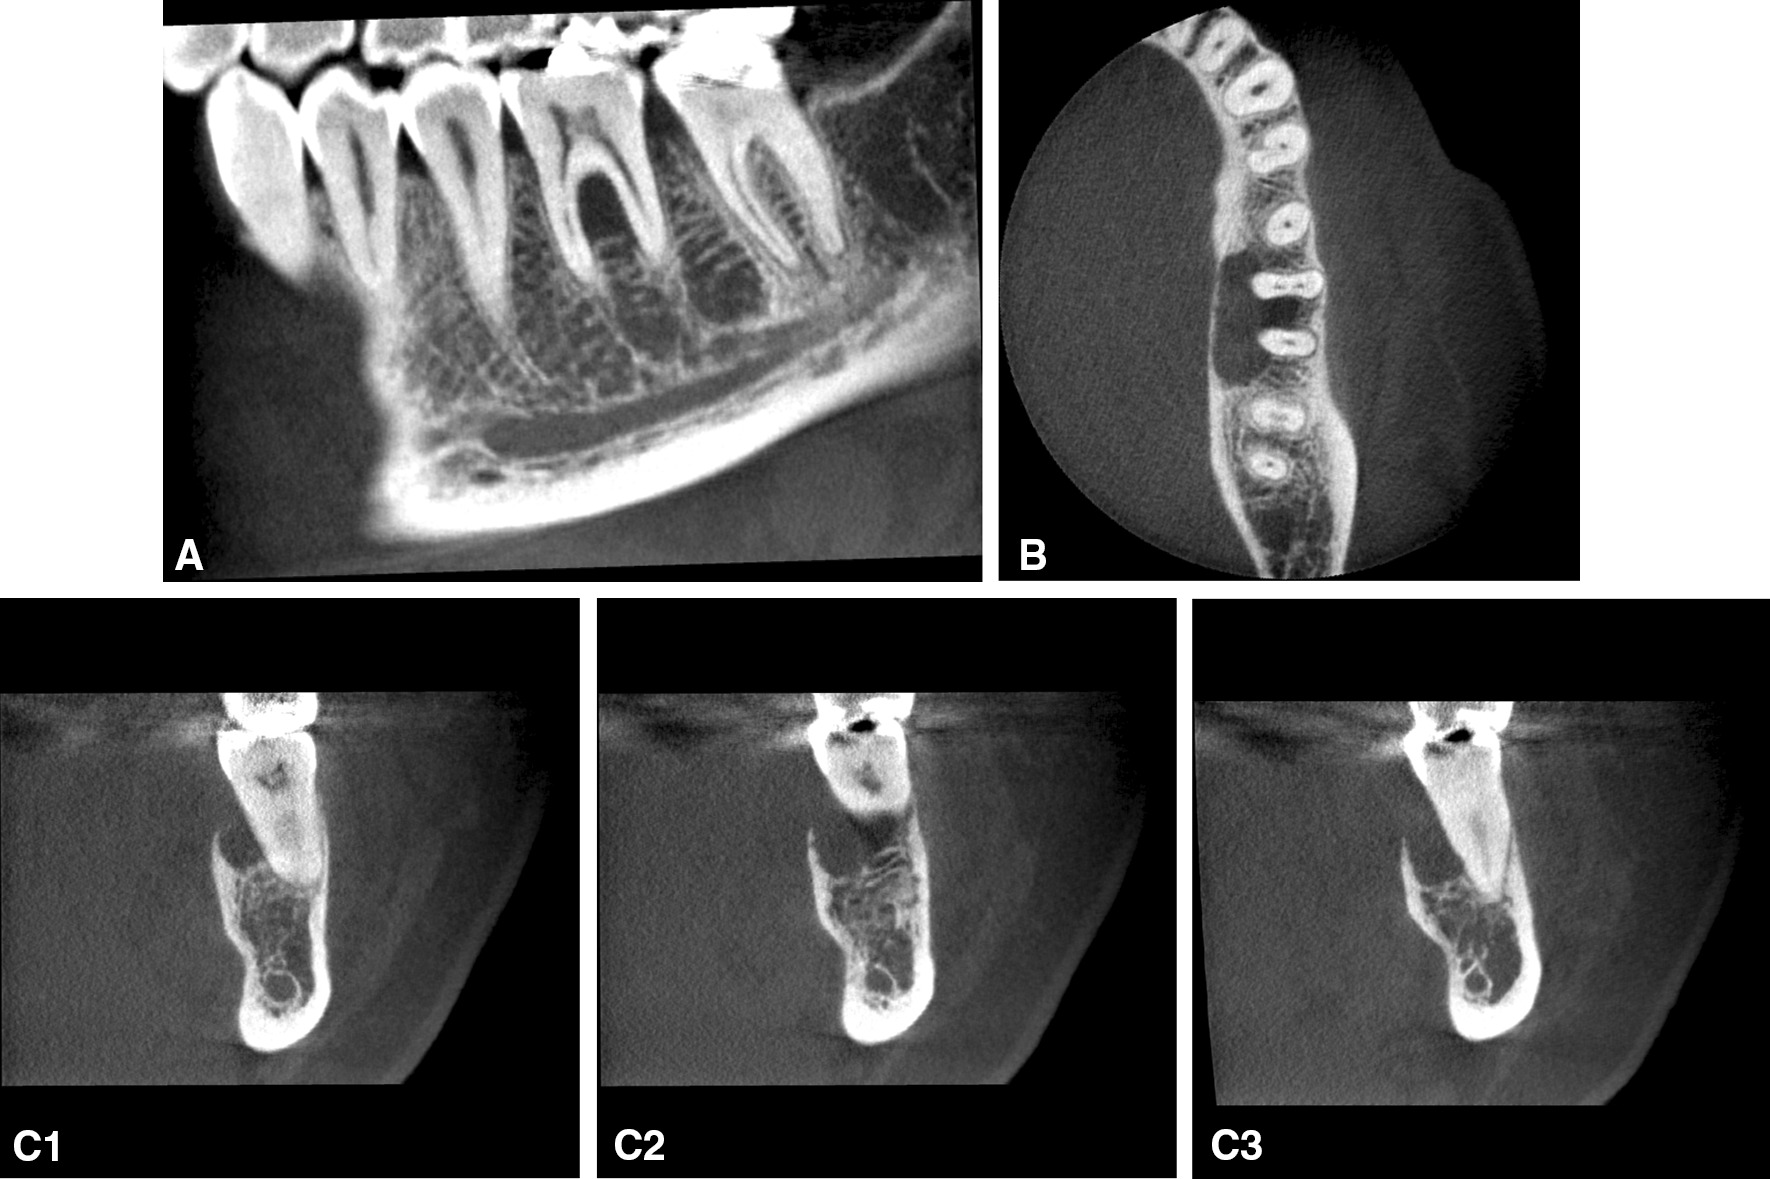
Cone beam computed tomography (Accuitomo 3D 170, Morita Corp, Kyoto, Japan) scan of the left mandible (full scan rotation 17.5 s, exposure setting 90 kV, 5.0 mA, field of view 6 × 4 cm). A radiolucency of the alveolar bone lingual and interradicular of the first left molar can be observed. The demarcation is partially unsharp and fenestrated to the crestal. (A: sagittal, B: axial, C1-3: coronal; C1: mesial root, C2: interradicular, C3: distal root)
